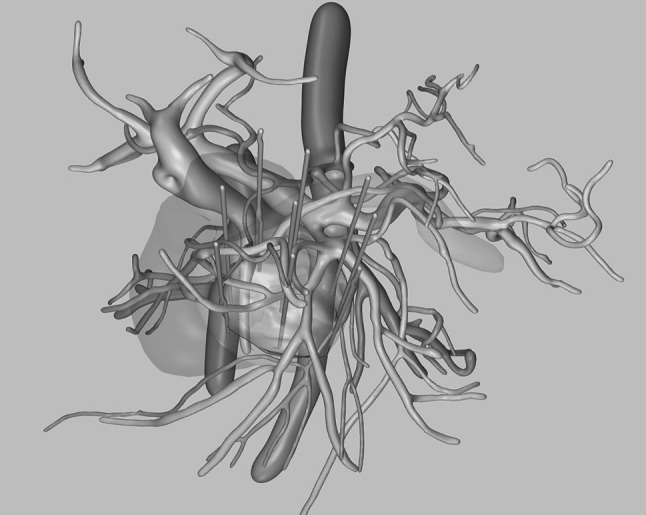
3D reconstruction of preoperative CT-scan for patient 3
